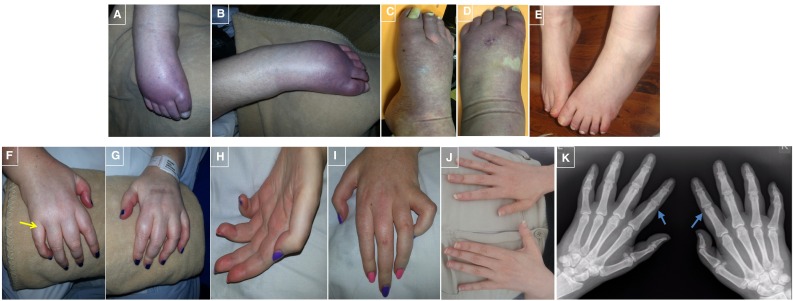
Photographs of the feet in SPG11 patients F1II:1 and F1II:2 (A and B) and F1AII:2 (C and D) showing peripheral oedema and marked discolouration of the feet that reverses with massage. Figure E are feet from a typical SPG11 patient in her mid 30s (Family 4, Tables 1, 2). Figure F to I shows photographs of the hands in the proband F1II:1 and F1II:2 showing generalized tapering of the fingers and swelling of the proximal phalanx, red discolouration of the hands, more marked in the palms. Figure J are hands from a typical SPG11 patient, Family 4. Figure K shows an x-ray of the hands (patient F1II:1) with mild narrowing of the proximal interphalangeal joints bilaterally (arrows) compatible with mild osteoarthritis
